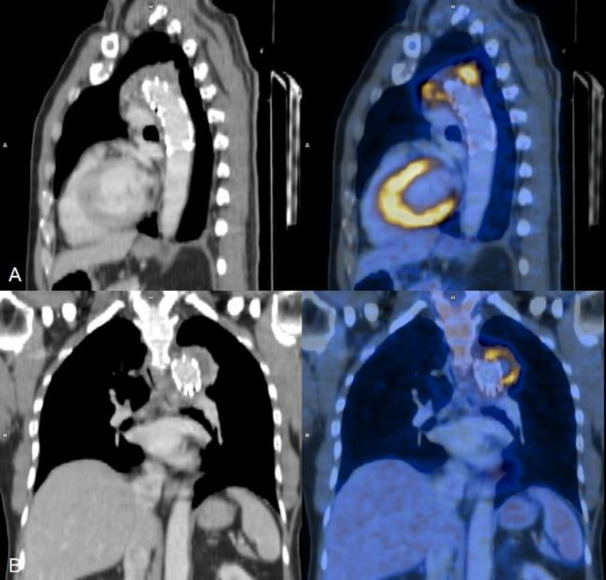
(A) Saggital and (B) Coronal section CT and fused FDG PET CT (left to right) images showing abnormal increased FDG uptake (SUV_Max_: 10.1) in aortic wall aneurysm in relation to the EVAR stent
